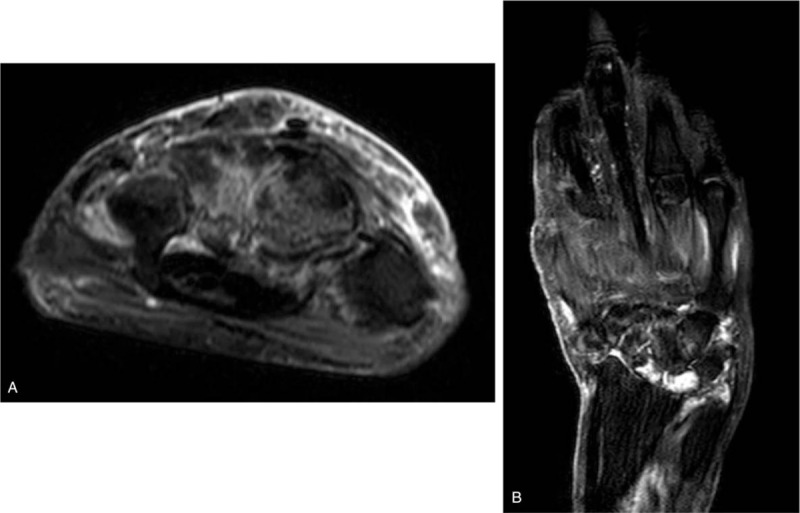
T2 weighted magnetic resonance imaging images at the 6-month follow-up. (A) Synovitis and fluid collection around the extensor tendons disappeared. (B) Signal intensity in the bone marrow of the carpal bones was improved.
